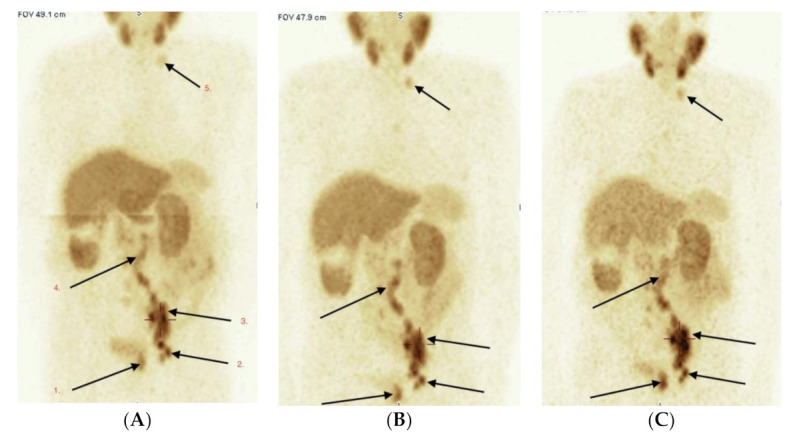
(A–C) Male 87 year, adenoca G3; GlS = 8 (4 + 4). PSA 228.0 ng/mL. Evaluation of tumor extent. Three scans of WB-SPECT/CT acquisition after 1 h (A), 3 h (B) and 6 h (C) after radio-tracer i.v. administration. Pathological high uptake of [99mTc]Tc-PSMA-T4 is seen in primary PCa—left lobe of prostate (1), multiple lymph nodes: left obturator (2), left external and common iliac (3), paraaortic left and right (4) and the left neck (5). No bone lesions were detected in current PSMA, bone and CT scans.
